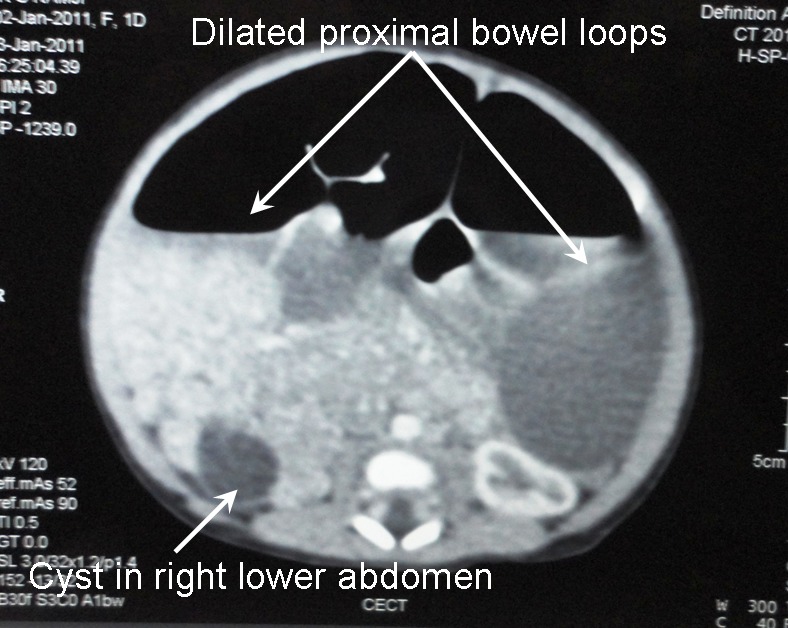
Figure 1: CT scan showing a cyst in the right lower abdomen with dilated proximal bowel loops.
